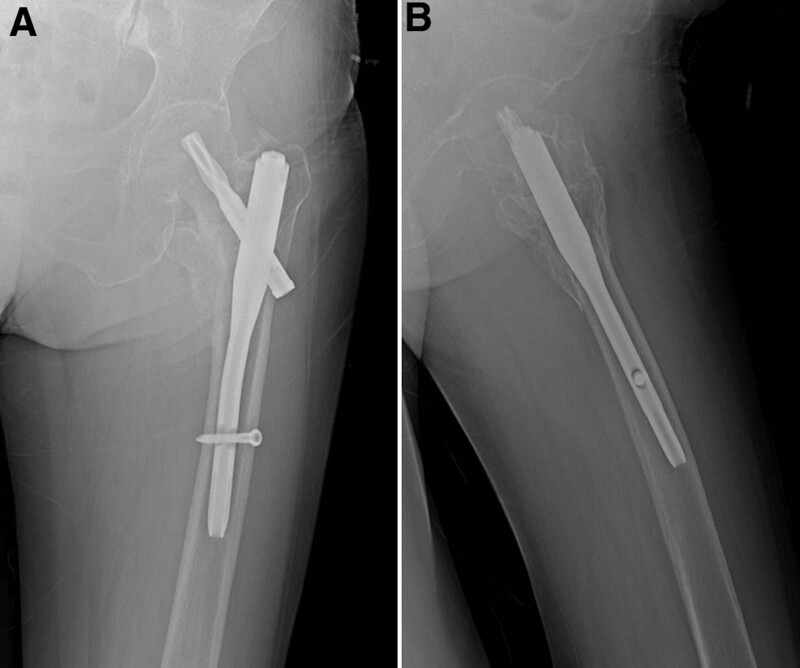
Radiographs of the left hip showed good fracture healing at 2-year follow-up. At 2-year follow-up, anteroposterior (A) and lateral (B) radiographs of the left hip showed good fracture healing.
